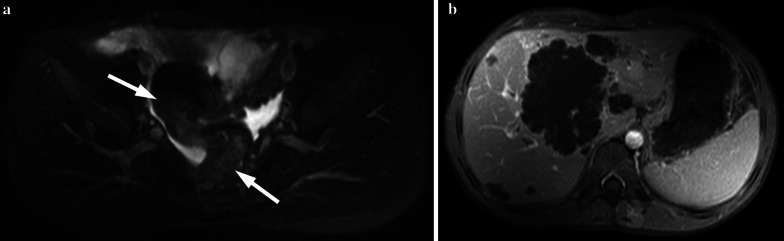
a, b A 14-year-old boy with colon mucinous adenocarcinoma. He had back pain and loss of appetite for the last 1.5 months. His father and uncle had colon carcinoma. a Axial fat-saturated T2-weighted image demonstrates diffuse increased wall thickening at the sigmoid colon (arrows). b Post-contrast T1-weighted image shows hypoenhancing liver metastases
